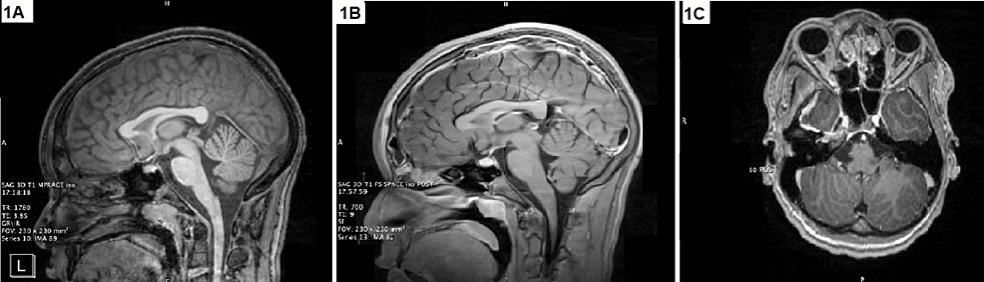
(A-C) Initial brain magnetic resonance imaging with early formation of a right frontal, maxillary, and ethmoid sinusitis and concerns for superior sagittal sinus thrombosis, small subdural empyema, and mild right frontal leptomeningeal enhancement
